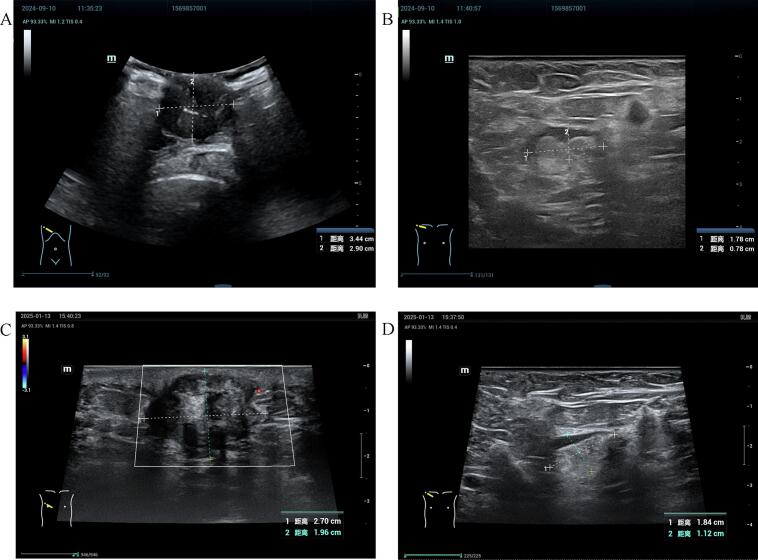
Ultrasonography of primary right breast mass (A) and right axillary lymph node (B) prior to neoadjuvant chemotherapy. Ultrasonography of the right breast mass (C) and right axillary lymph node (D) post-neoadjuvant chemotherapy.
